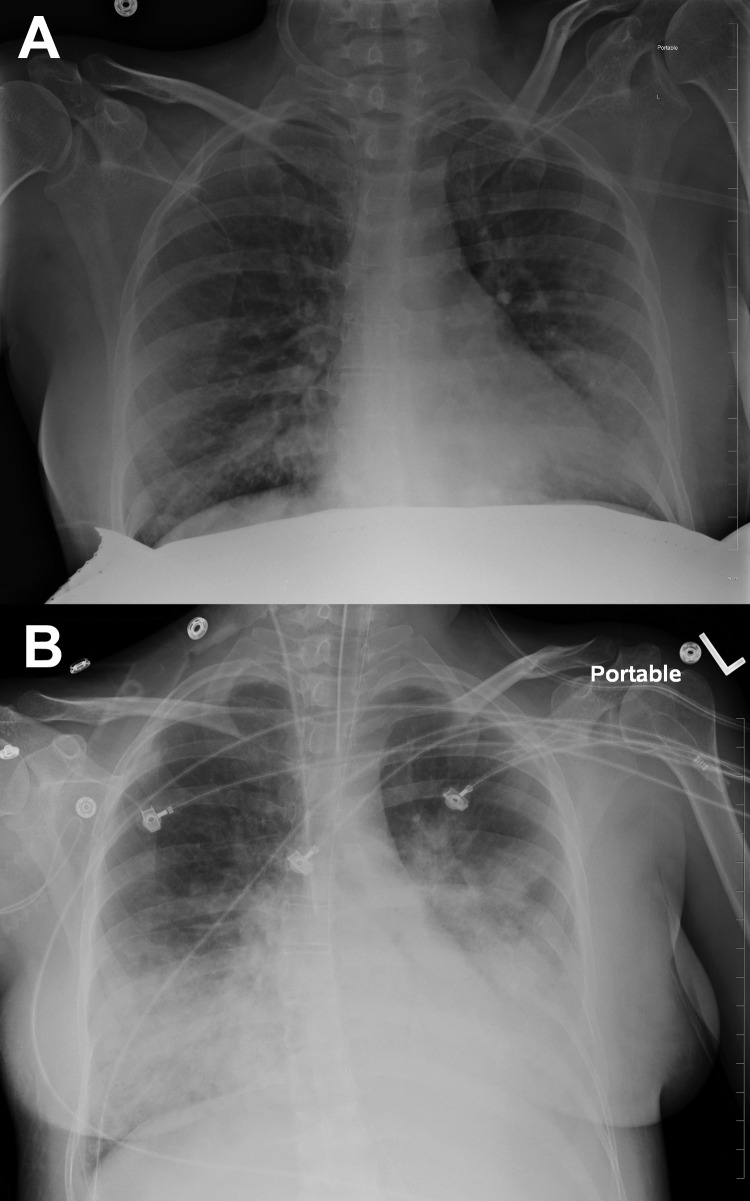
Course of influenza B virus infection and necrotizing pneumonia in peripartum woman, 2012, New York, USA. A) Chest radiograph at time of admission. B) Chest radiograph 1 day later, demonstrating progression of pneumonia.
